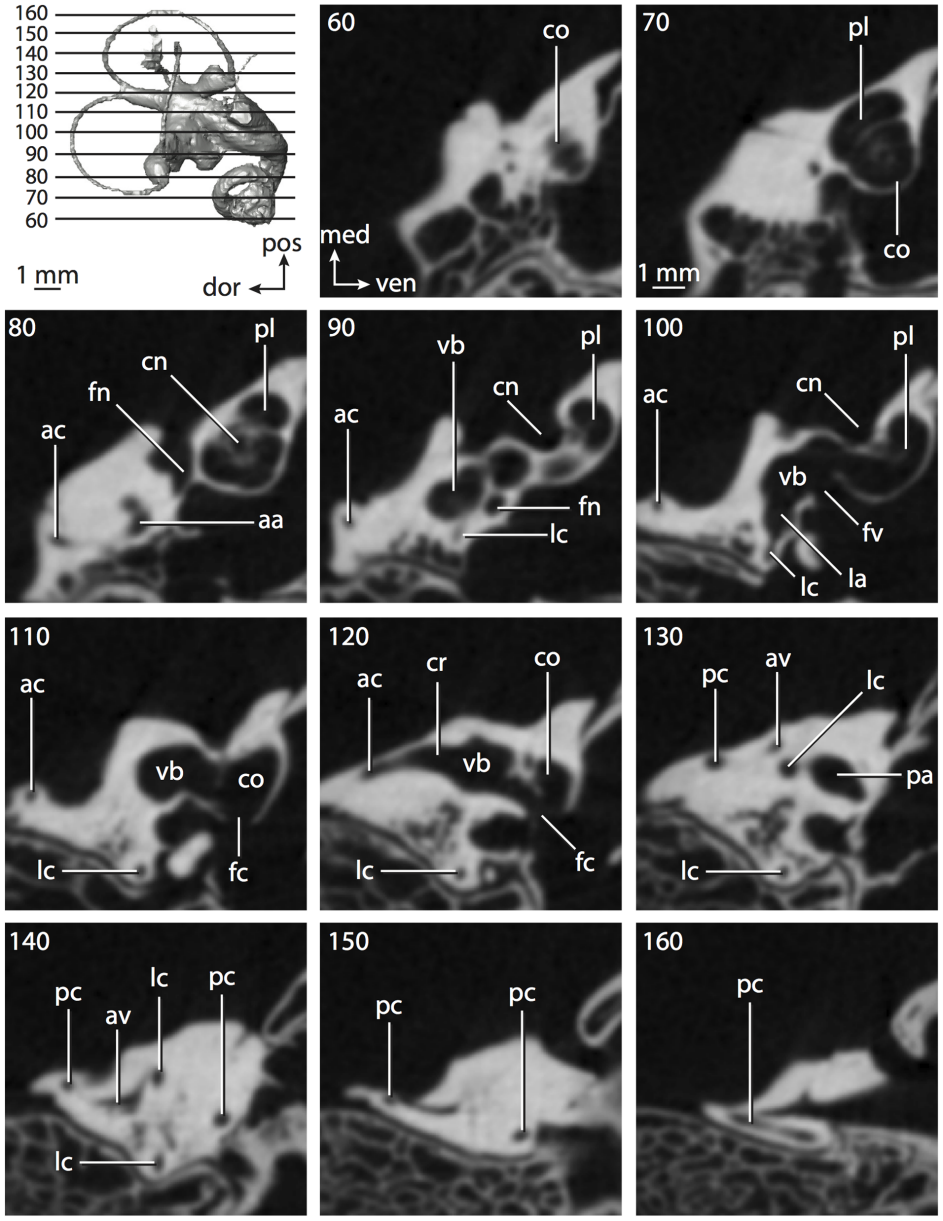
CT slices through ear region of*Procavia capensis*. Abbreviations listed at the end of the Materials and Methods section.
